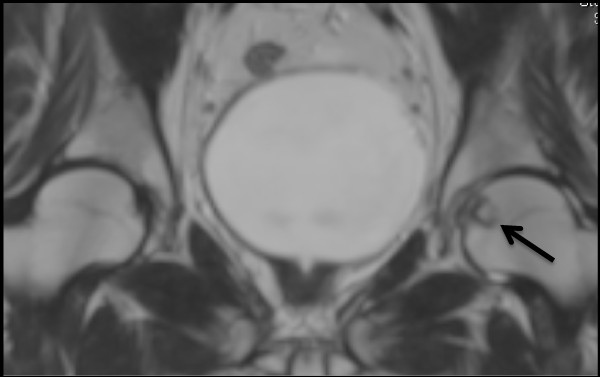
T2-weighted coronal image showing hyperintense focus with a hypointense rim (black arrows).
